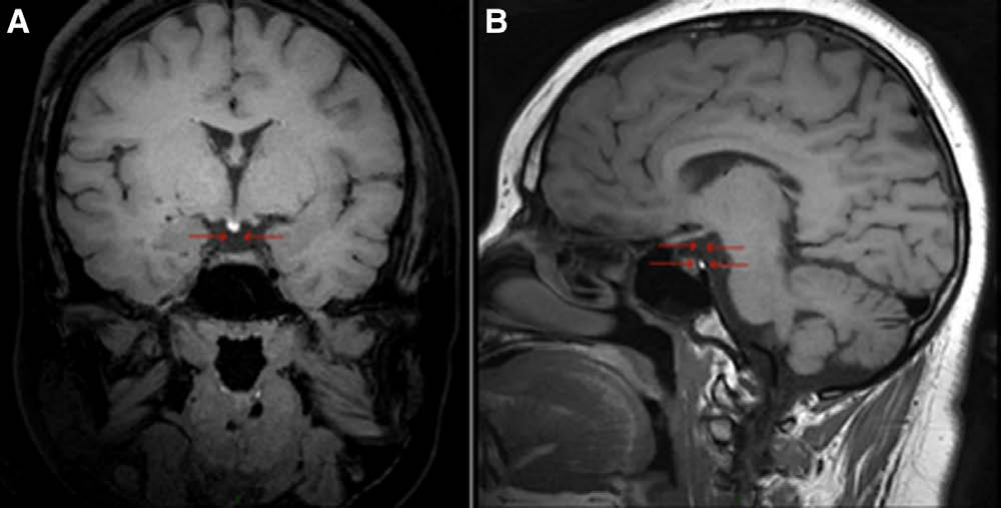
Pituitary stalk interruption syndrome/pituitary stalk agenesis/pituitary posterior lobe ectopic: MRI scan of the pituitary gland (A: coronal, B: sagittal): The pituitary volume is diminished, with a pituitary height diameter measuring approximately 3 mm. The pituitary stalk is absent, and there is ectopia of the posterior lobe of the pituitary. MRI = magnetic resonance imaging.
